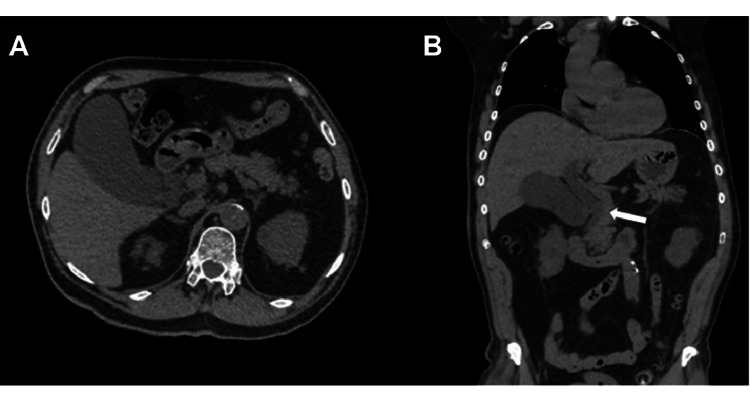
Abdominal CT findings A) Axial CT image demostrating gallbladder hydrops. B) Coronal CT image evidencing compression of the common bile duct by the infundibulum and retrograde dilation of the duct.
